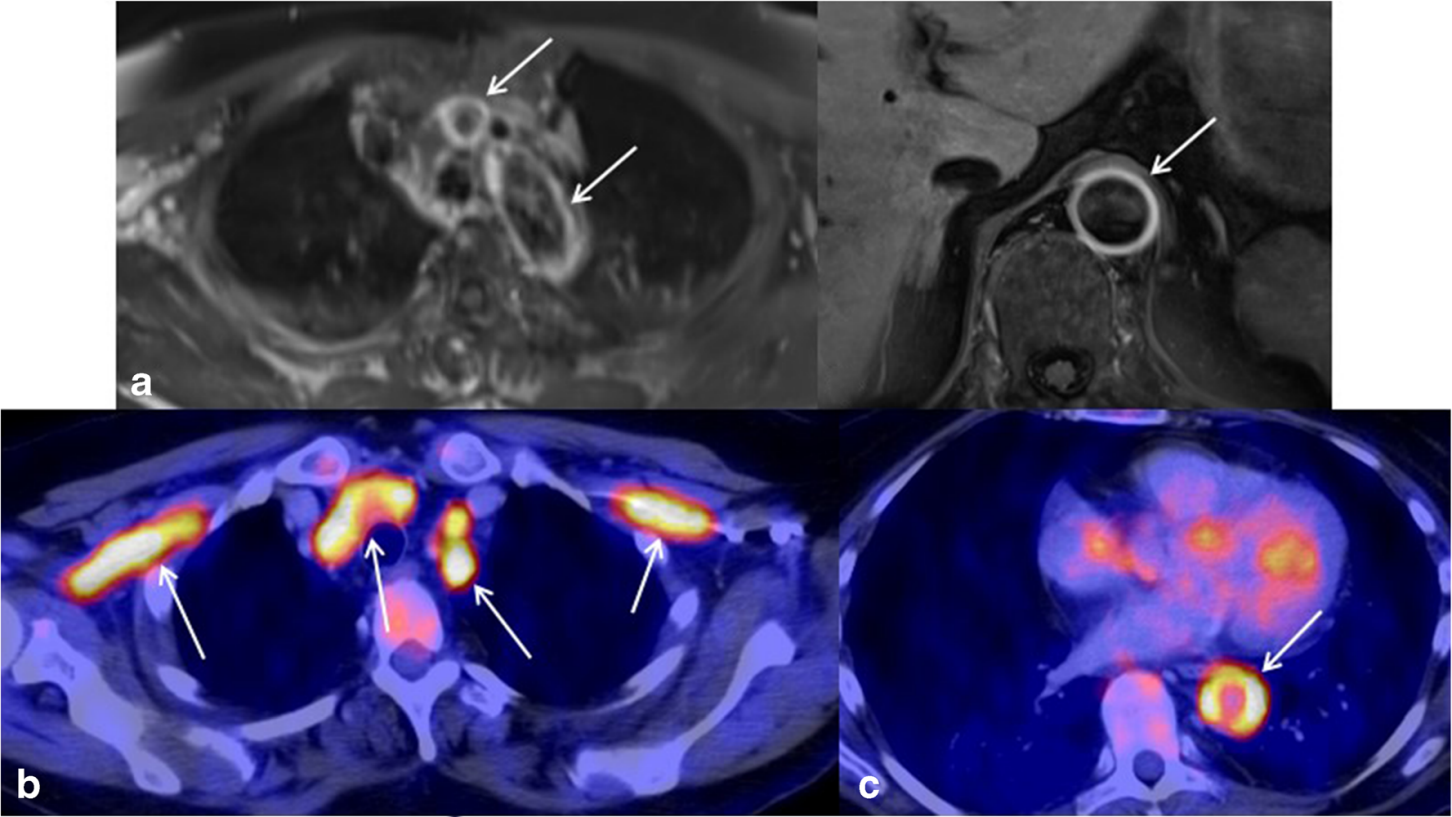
Characteristic findings in GCA with involvement of the aorta and first major branches: T1-weighted MRI shows smooth concentric vessel wall thickening and enhancement of the thoracic and abdominal aorta and the brachiocephalic trunk (a, b, white arrows). FDG-PET-CT reveals a correlating significant tracer uptake of the inflamed vessel walls along the thoracic aorta (d, white arrow) as well as along the supra-aortic branches including the bilateral axillary artery (c, arrows)
